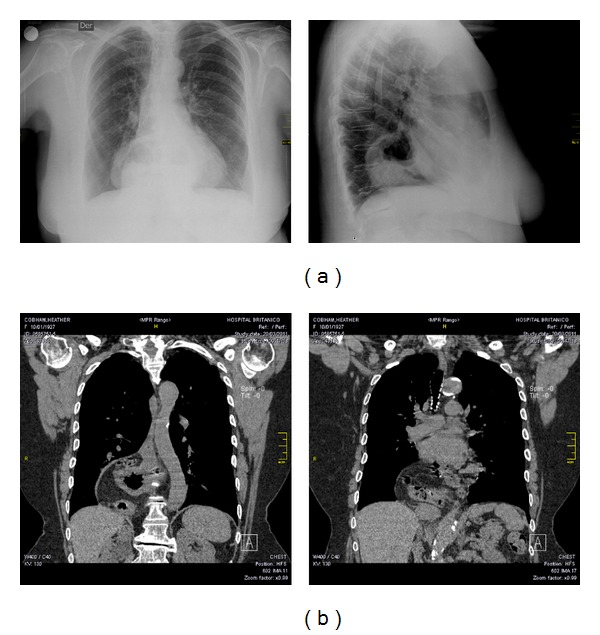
(a) A large retrocardiac opacity is observed with air and liquid level compatible with a giant hiatus hernia. (b) A CT-scan with reconstruction is shown validating the diagnosis. The mass corresponding to the stomach and likely small bowel is located behind the heart and in close relation to the left atrium.
